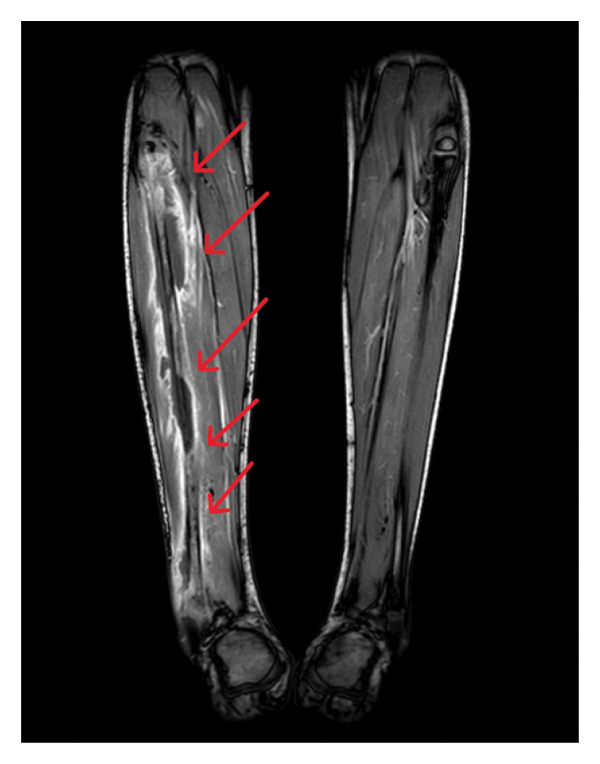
MRI of the lower limbs (T1‐weighted postcontrast) demonstrating a multiloculated rim‐enhancing fluid collection surrounding the right fibular diaphysis, extending craniocaudally for approximately 27 cm with a maximal thickness of 1.5 cm. Associated periosteal elevation with reactive periosteal enhancement is noted. Subtle cortical erosions are present along the medial and posterior aspects of the proximal fibular metaphysis.
